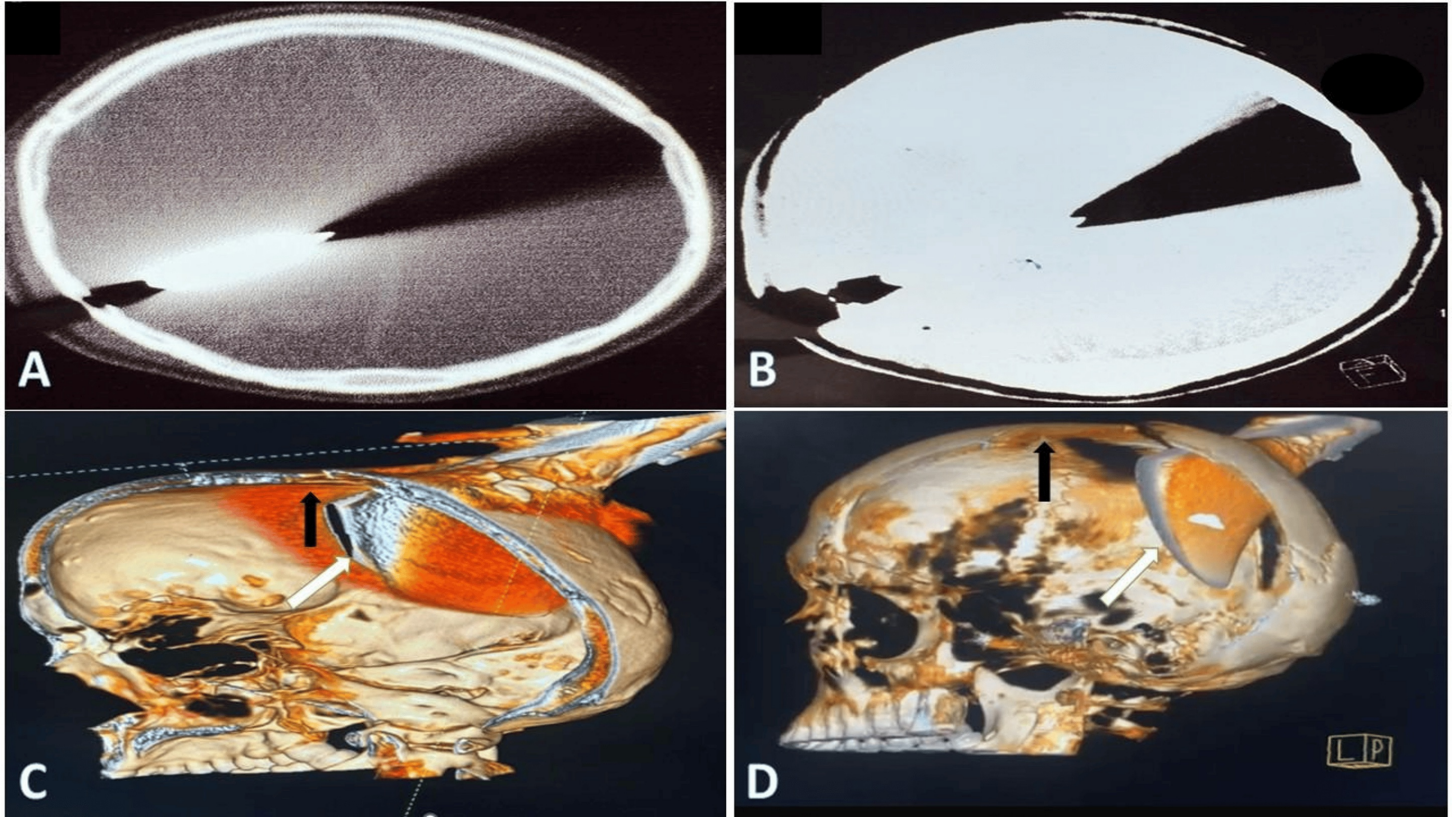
Preoperative computed tomography (CT) and three-dimensional reconstruction demonstrating the intracranial trajectory of the retained axe. (A) Axial CT scan (brain window) showing the metallic foreign body penetrating the right parietal bone with associated streak artifact.
